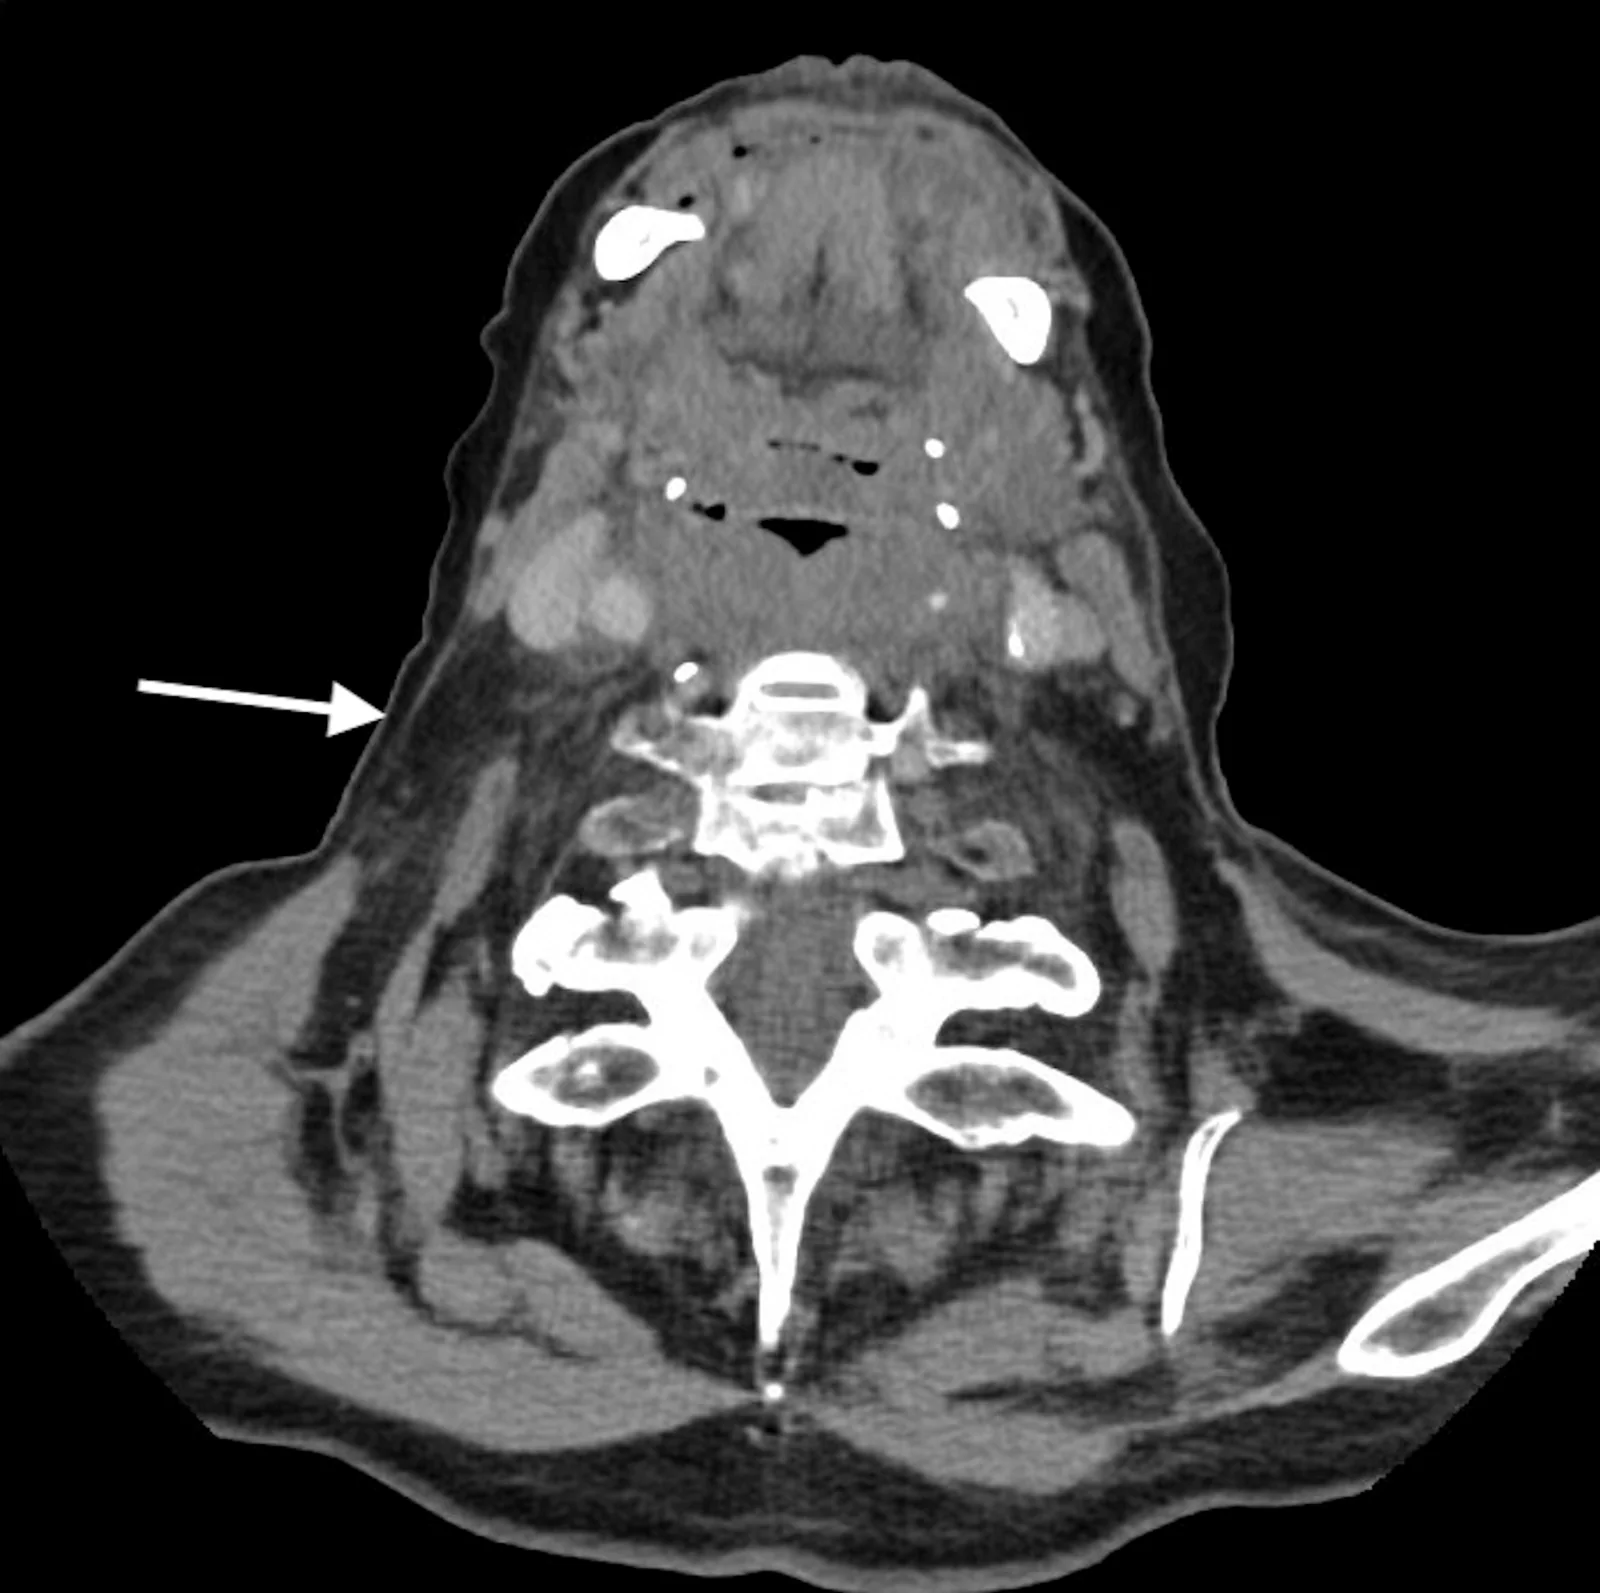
CT image of the neck demonstrating a hematoma within the right sternocleidomastoid muscle.
